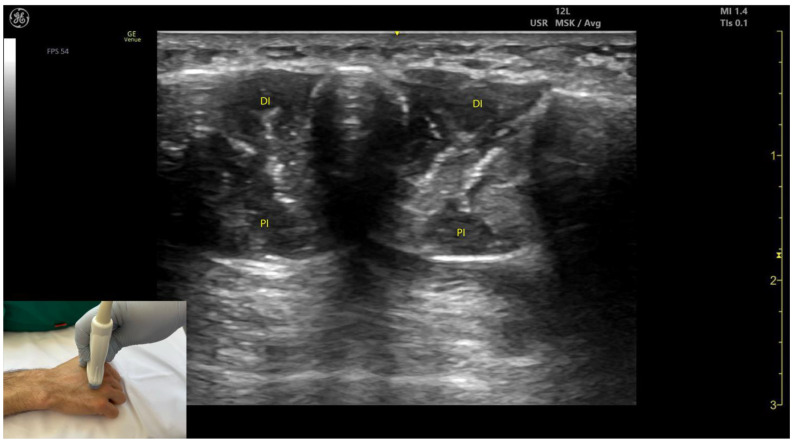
Ultrasound anatomy of the interossei muscles with key landmarks: DI—dorsal interossei; PI—palmar interossei.
